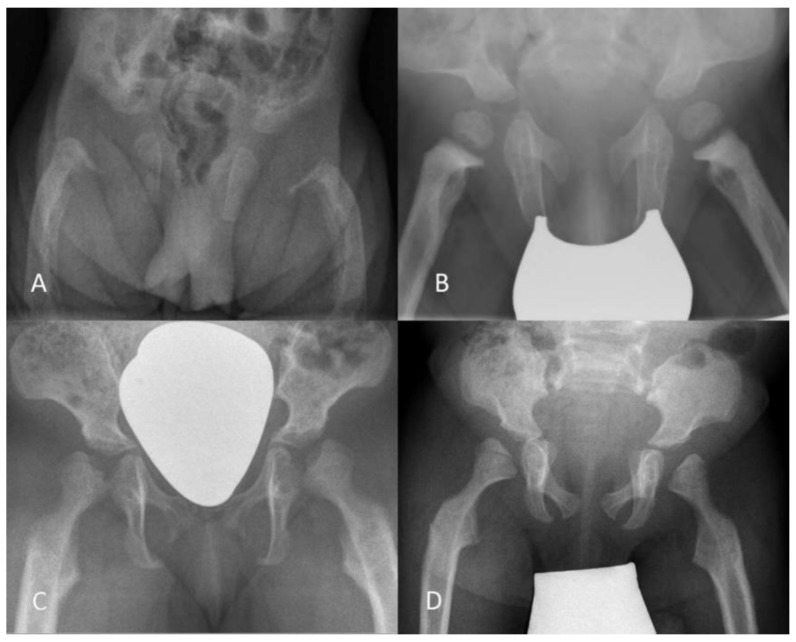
Qualitative radiographic assessment. (A) Rickets/hyperparathyroidism-like changes with metaphyseal irregularities, periosteal cloaking and femoral bowing in a male MLII patient at 1 week of age (*n* 11). (B) Coxa valga with widening of the growth plate of the proximal femur in a male MLII patient at 1 year of age (*n* 5). (C) Osteonecrosis–like changes of the femoral head, flared iliac wings in a female ML intermediate patient at 8 years of age (*n* 13). (D) Constriction of the femoral neck with medial flattening of the epiphysis in a male ML intermediate patient at 9.3 years of age (*n* 15). (B–D) Constrictions of the supra-acetabular part of the os ilium.
